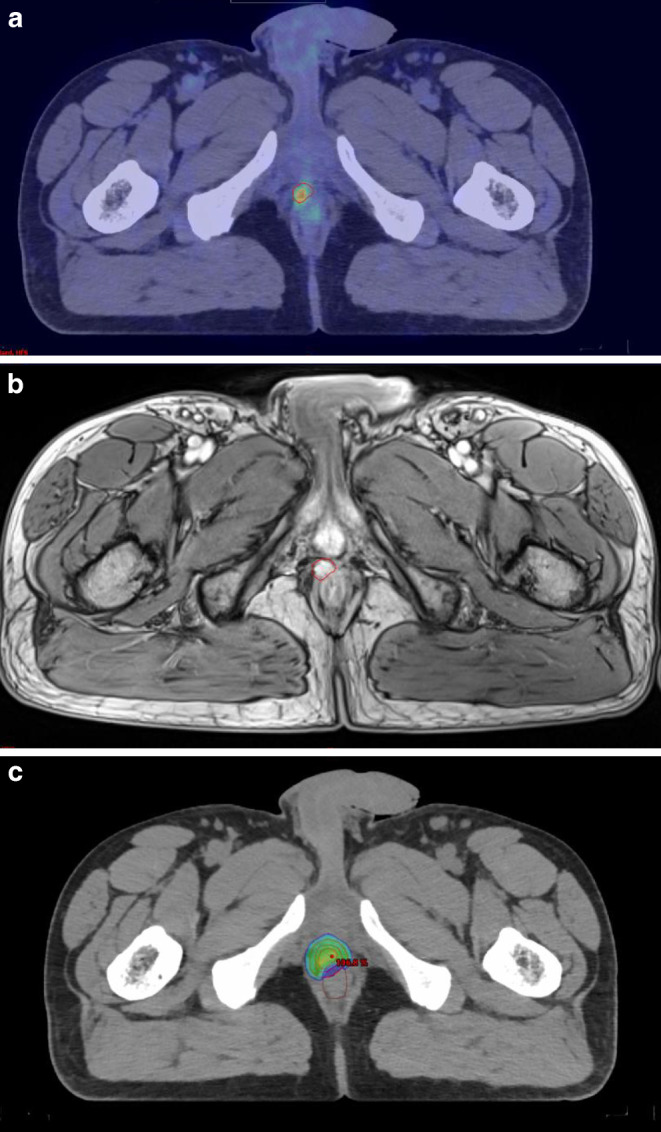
Imaging and dose distribution in an exemplary patient with local recurrence. a PSMA-PET: *red line* GTV, b MRI: *red line* GTV, c Dose distribution: *inner red* GTV, *middle red* CTV, *outer red* PTV, *brown* rectum
